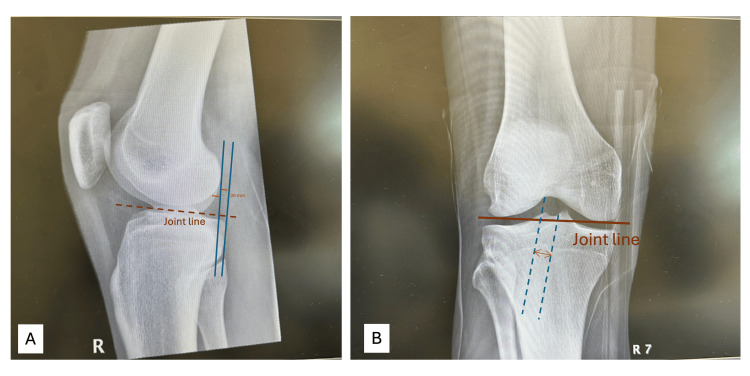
Preoperative X-ray radiographs demonstrating posterior tibial translation (A) Lateral view demonstrating posterior subluxation of the tibia relative to the femur, with a measured posterior translation of approximately 20 mm. (B) Posterior stress AP view showing posterior tibial translation under loading, consistent with posterior instability and isolated PMB rupture. PMB: isolated posteromedial bundle, AP: anteroposterior
